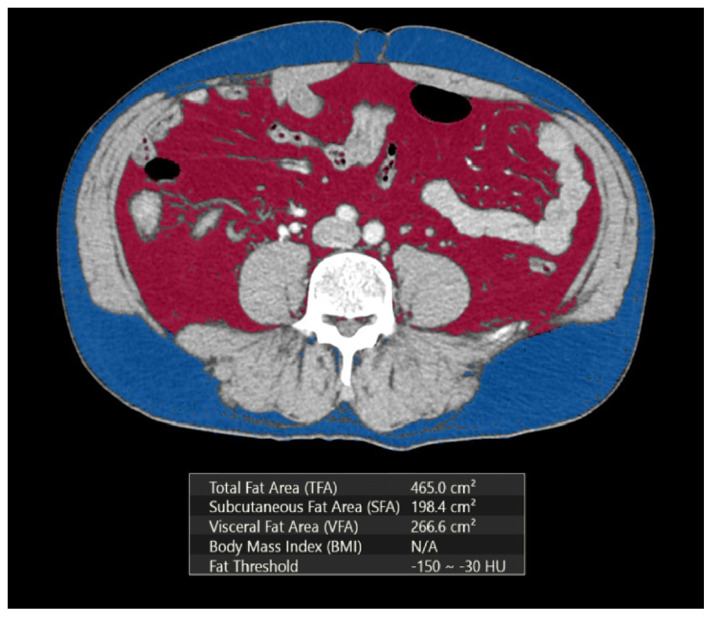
Automatic calculation of visceral and subcutaneous fatty tissue in the range of −150 and −30 HU on the CT image in the axial plane at the level of the umbilicus.
